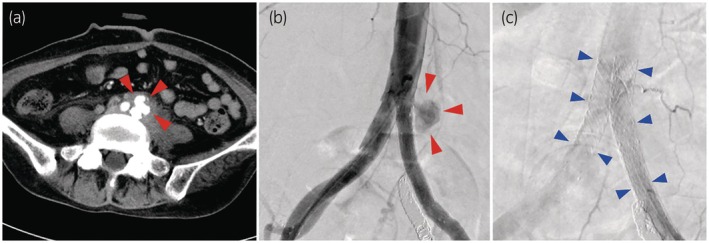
(a) CT angiography shows contrast spillage at the left proximal CIA (red arrows) at the proximal end of the stent graft placed for the left UAF 1 year before. (b) Angiography shows a left CIA aneurysm (red arrows). (c) Implantation of stents (blue arrows) in the bilateral CIA using the so‐called kissing stent technique, with a slight overlap within the distal aorta to create a “kissing” configuration at the aortic bifurcation. This technique allows endovascular treatment of lesions involving near the aortic bifurcation.
